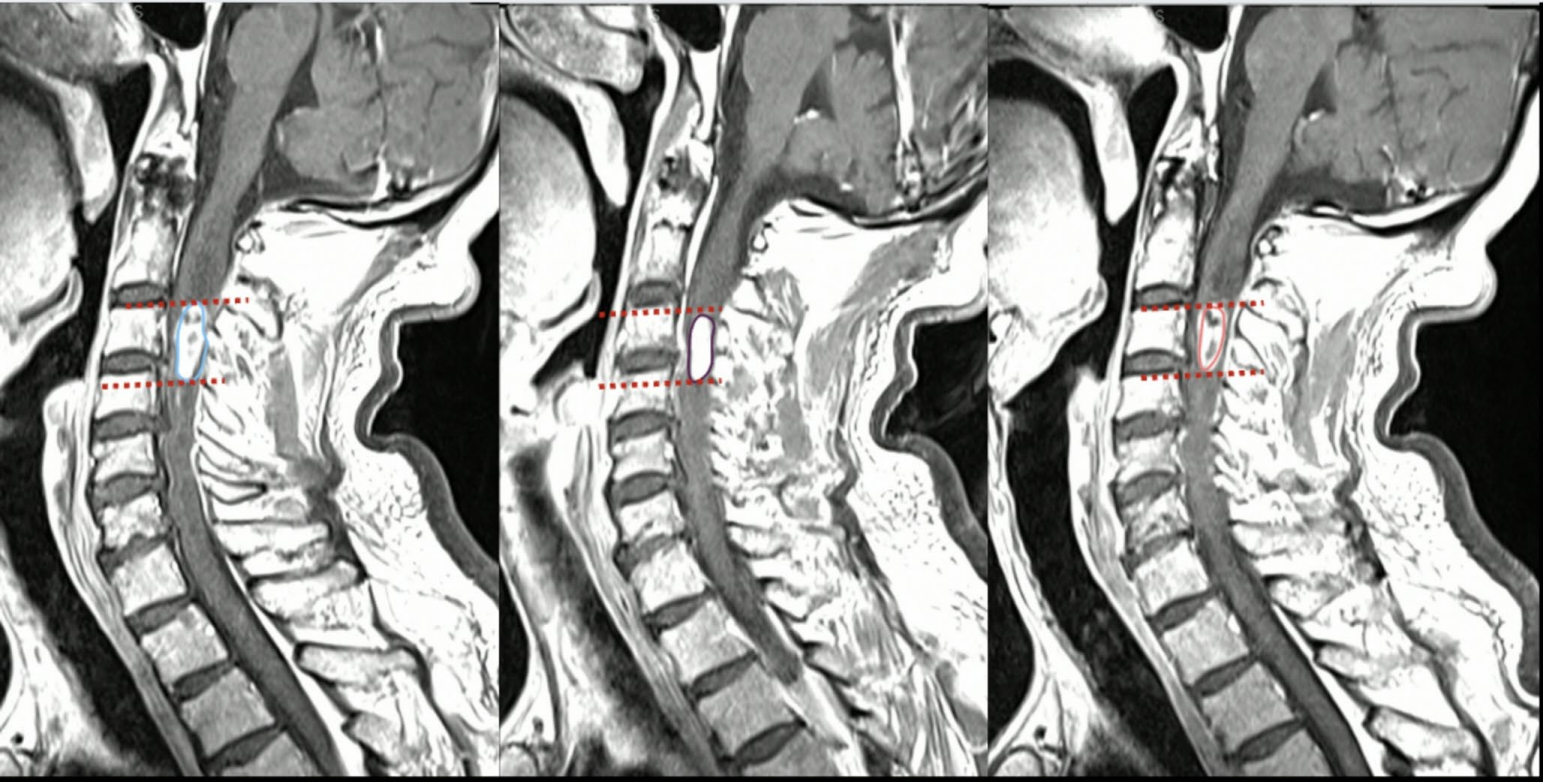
Sagittal T2-weighted MRI images of the cervical spine showing three consecutive slices from the same patient. An intradural extramedullary tumor is visible in all three slices. Tumor tissue was manually segmented and annotated (outlined in red). The lesion was confined to a single vertebral segment in cranio-caudal extension
